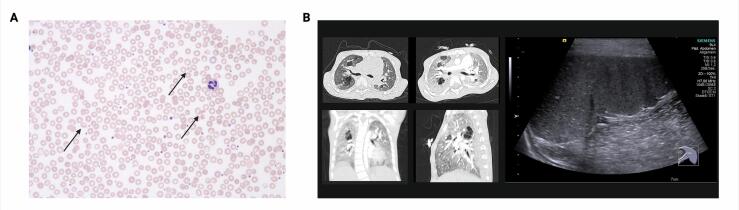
Clinical presentation of HO-1 deficiency. A. Mild changes of erythrocyte morphology were observed in peripheral blood smears. Fragmentocytes with helmet and crescent shapes are shown by arrows. B. Lung CT scans showed prominent structural changes, including bullae, bipulmonal peribronchiovascular thickening, and bipulmonary consolidations. In contrast to previously reported cases, splenomegaly was observed by ultrasound examination.
